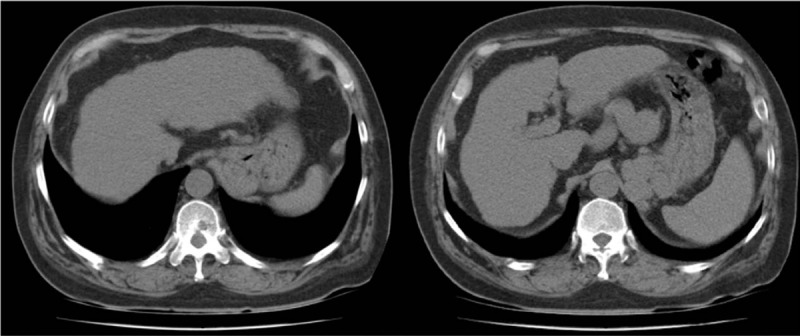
Abdomen CT on Dec 5, 2018. It revealed liver cirrhosis with atrophy of liver, fat deposition in liver, esophageal varix along lesser curvature of stomach and no ascites.
